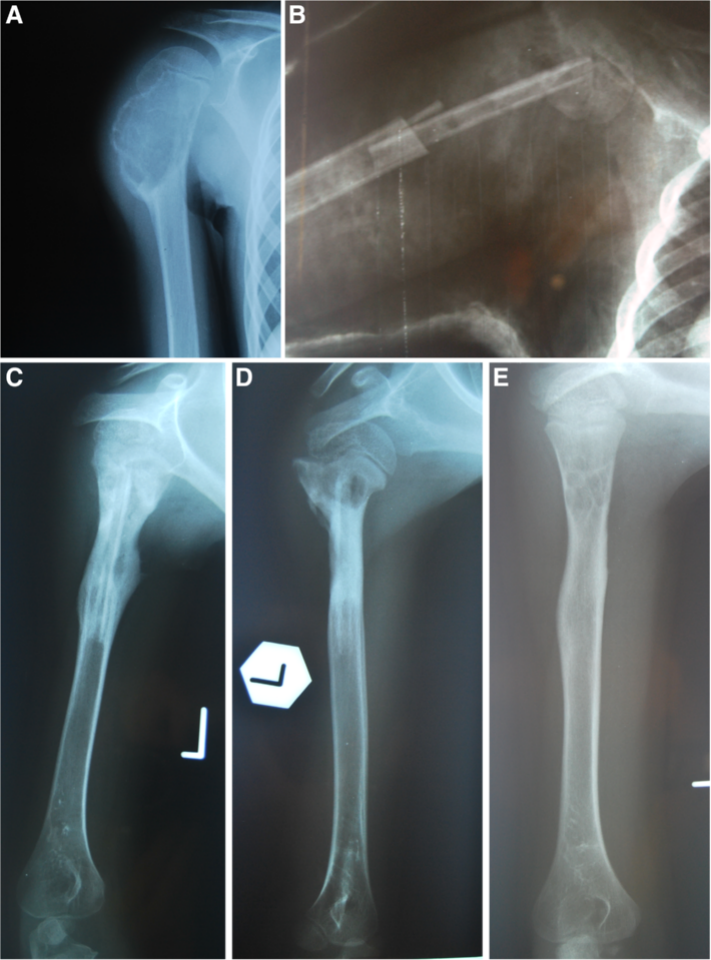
Example of a patient with an aneurysmal bone cyst (ABC) in the humerus treated with en bloc excision of the affected bone fragment and autogenous fibular graft reconstruction. (A) Preoperative X-ray of the lesion; (B) X-ray follow-up directly after the surgical procedure; (C) at 3 months postoperatively; (D) at 6 months postoperatively; and (E) final result, 3 years postoperatively. The ABC is healed with small cystic lesions.
